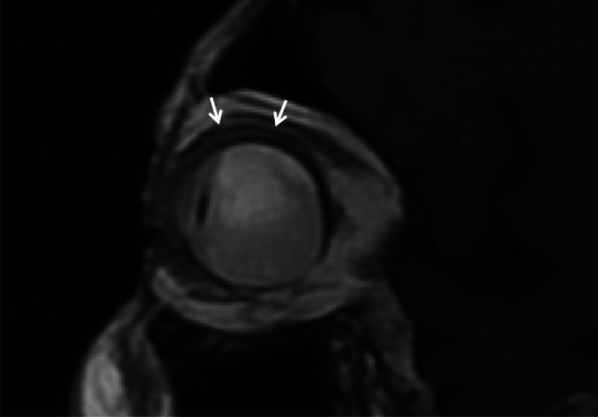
An 82-year-old man with radiation-induced periocular fibrotic adhesions after brachytherapy for choroidal melanoma of the right eye. The same patient as in Fig. 7. Sagittal T1-weighted image shows soft tissue thickening along the superior outer edge of the sclera (white arrows), a finding consistent with fibrotic adhesions
